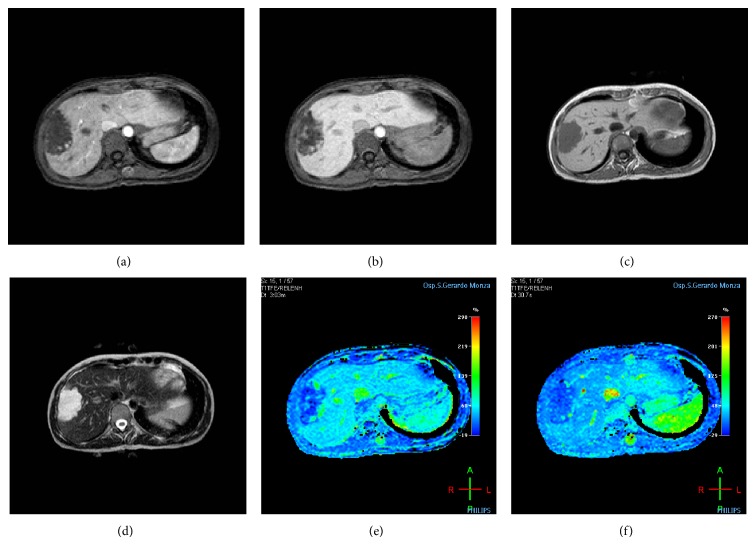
A 44-year-old man with gallbladder stones was evaluated. The MR imaging shows a focal lesion in the right lobe. (a, b) Axial arterial and delayed postcontrast injection images show irregular well-defined lesion, characterized by globular pattern enhancement at the periphery of the lesion in the arterial phase, with intensity signal that increases during delayed phase. (c) Axial T1-weighted in-phase echo-gradient sequence shows a homogeneous hypointense well-defined irregular nodular area in the seventh segment. (d) Axial T2 fat-sat weighted sequence shows well-defined nodular area, with high intensity signal. (e, f) On colour perfusion maps (Relative Arterial Enhancement and Relative Late Enhancement, resp.) the lesion corresponds to hypovascularized area, characterized by high vascularity hot-spots signals along the boundary of the lesion.
